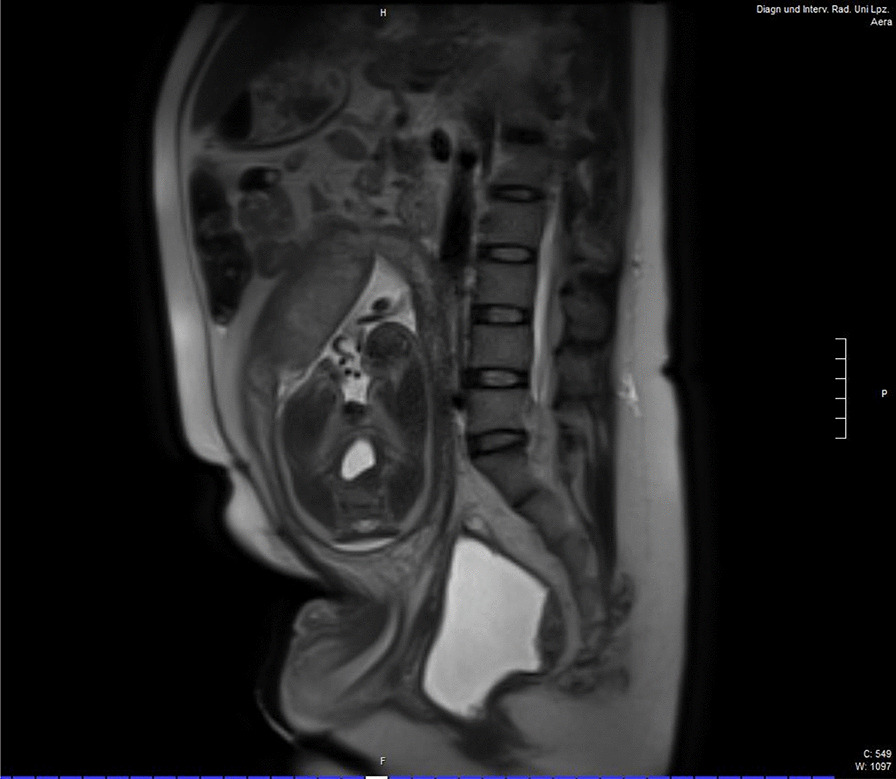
Magnetic resonance imaging (MRI) of the patient at 34 weeks gestational age showing the exact position of the uterus and the fetus in breech position
